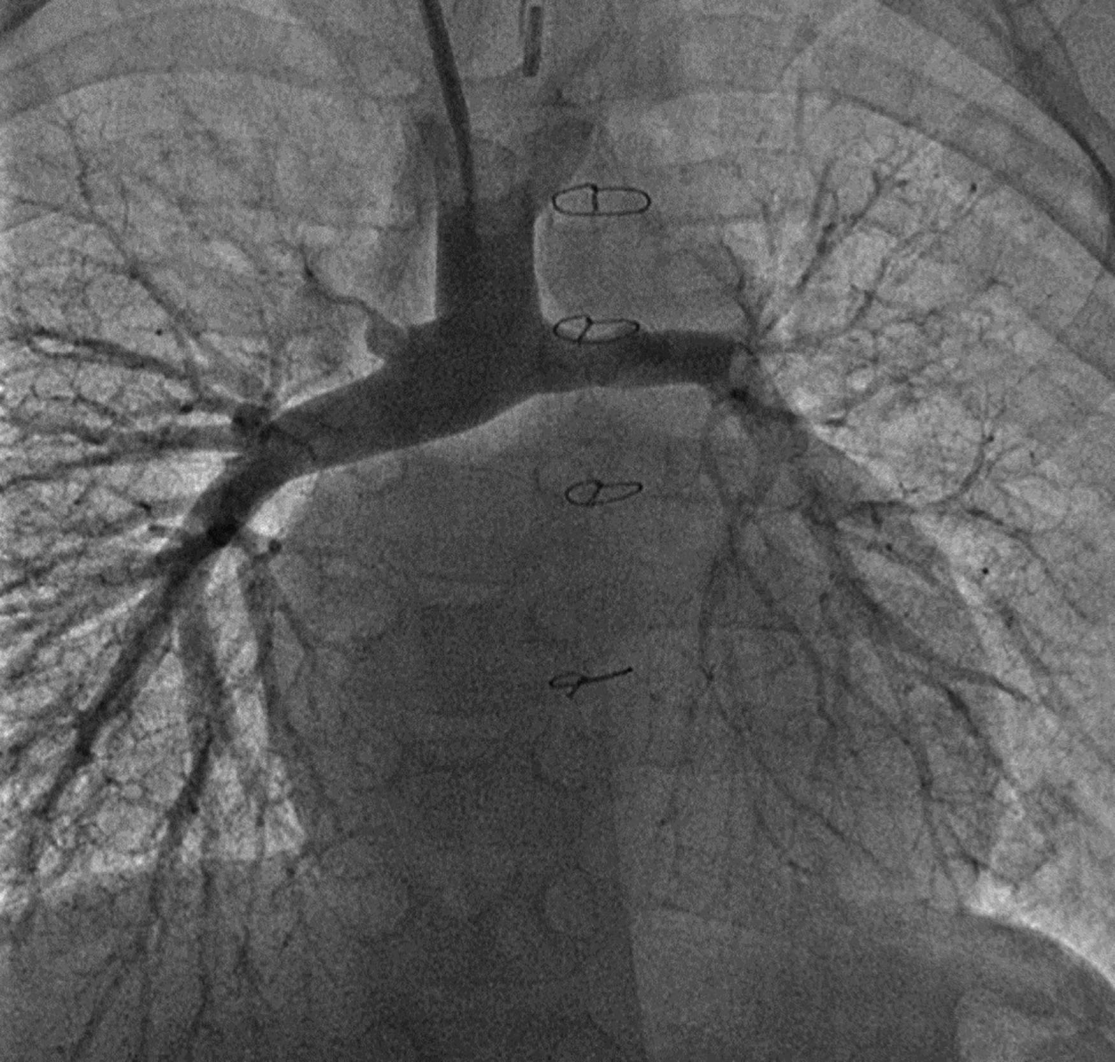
His post-TCPC course was complicated by a large chylous left pleural effusion confirmed by ultrasound (Figure 2) on POD 6. Echocardiogram (ECHO) demonstrated mild RV systolic dysfunction, mildly dilated right atrium and RV, mild TR, and mild neo-aortic insufficiency and no fenestration. Pulmonary edema and increased Fontan pressures (17–21 mm Hg) persisted despite diuretics and milrinone infusion. He subsequently developed methicillin resistant staphylococcus aureus (MRSA) sepsis and tricuspid valve endocarditis requiring intubation and escalation of vasoactive infusions, and ultimately peripheral cannulation for veno-arterial extracorporeal membrane oxygenation (VA ECMO) POD 10–11. At the time of cannulation Fontan pressures were 23–25 mmHg and he had a moderate-severely dilated RV with moderate-severely decreased RV systolic function, and moderate TR by ECHO. He was successfully decannulated after 7 days of ECMO. Fontan pressures were 21–28 mmHg following decannulation, a vegetation remained visible with persistent moderate TR. Further ECHO data is referenced in Table 1.
